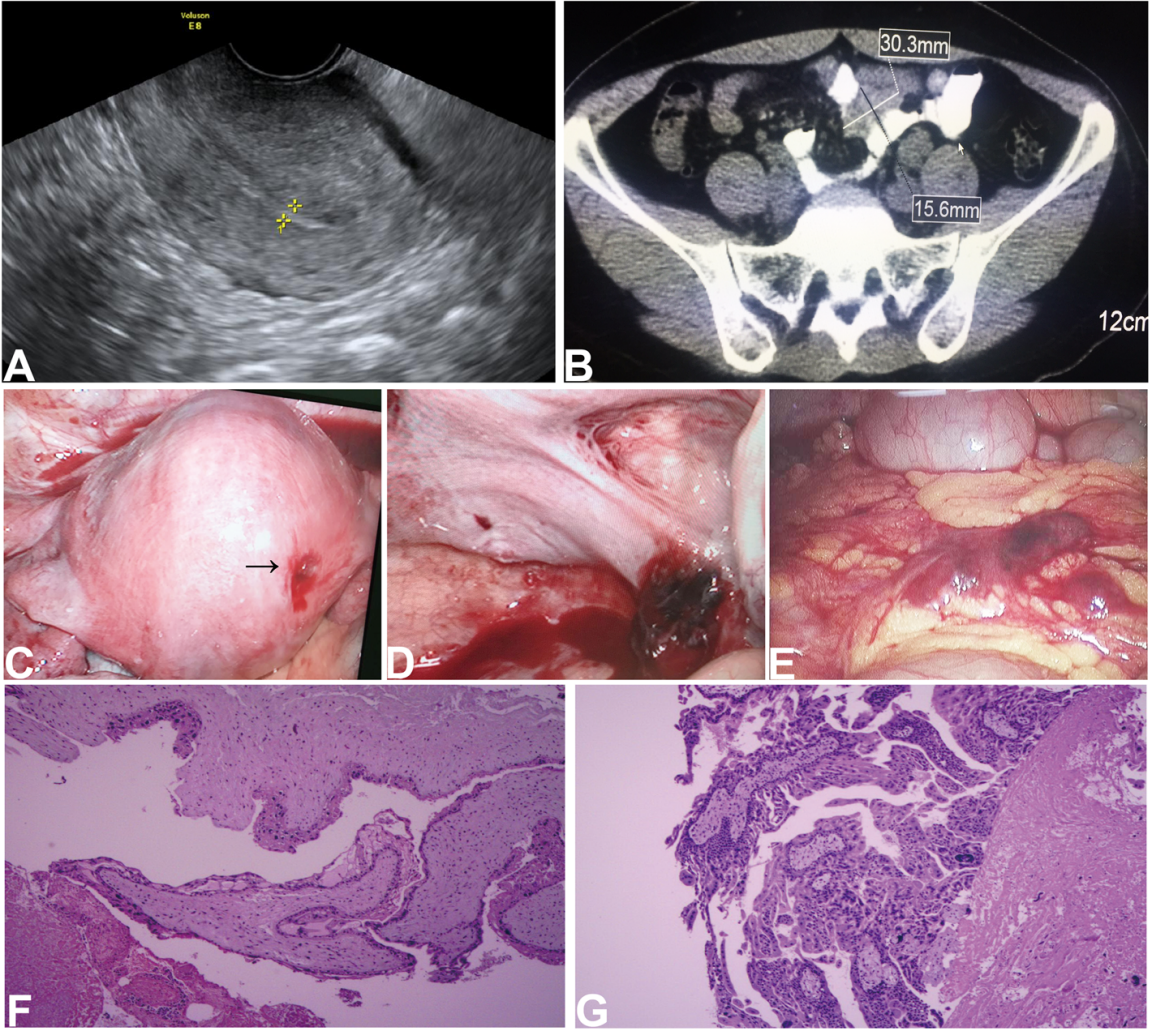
a-b An ultrasound examination (a) and a CT scan (b) of the uterine and pelvis. c-e An iatrogenic uterine perforation (arrow) (c) and the lesions [right uterosacral ligament (d) and omentum (e)] of mole under laparoscopy. f-g Edematous villus with trophoblastic proliferation was significant in omentum (f) and right uterosacral ligament (g)
